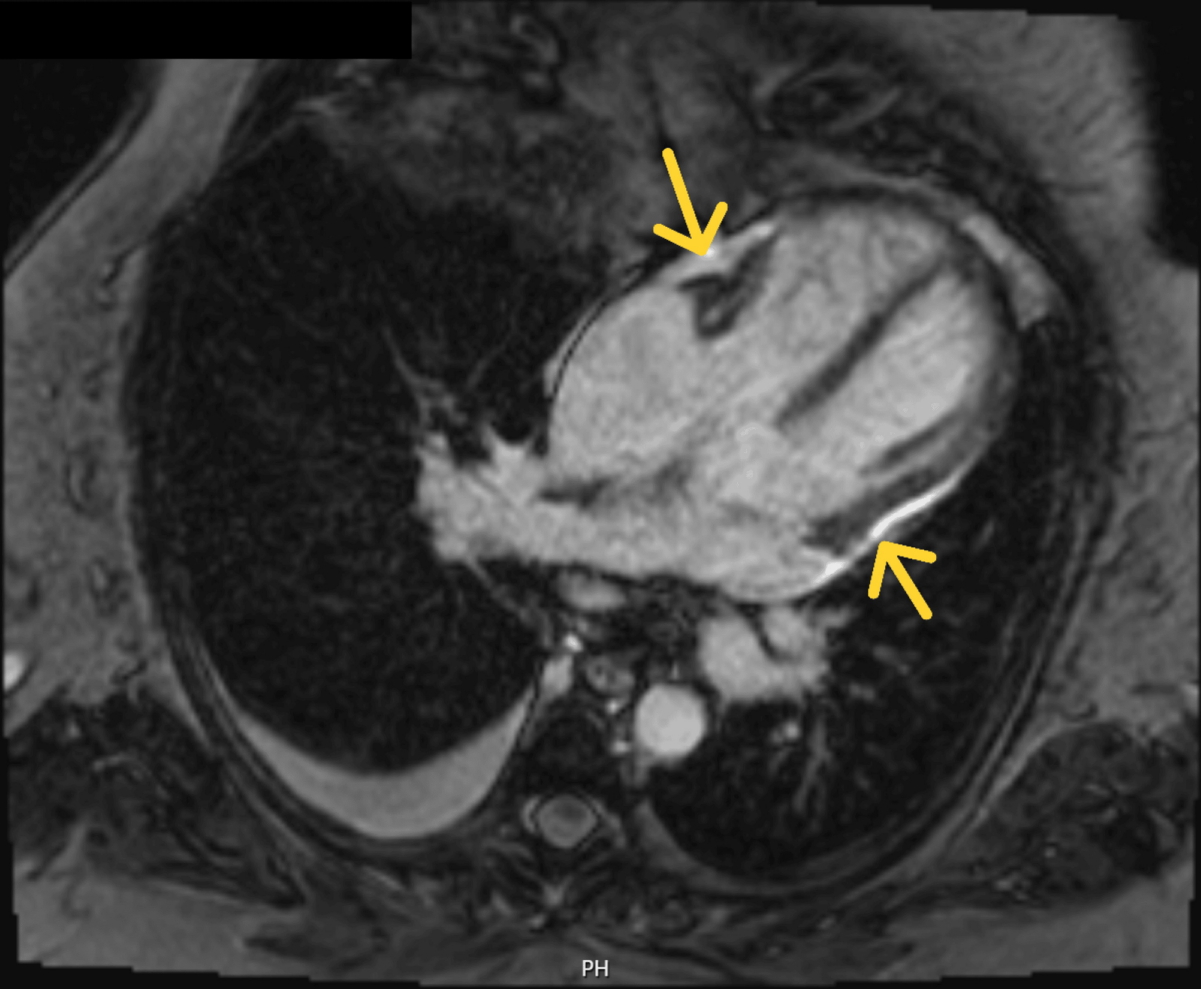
Cardiac MRI apical four-chamber view showing pericardial thickening and late gadolinium enhancement of the pericardium (yellow arrows)
